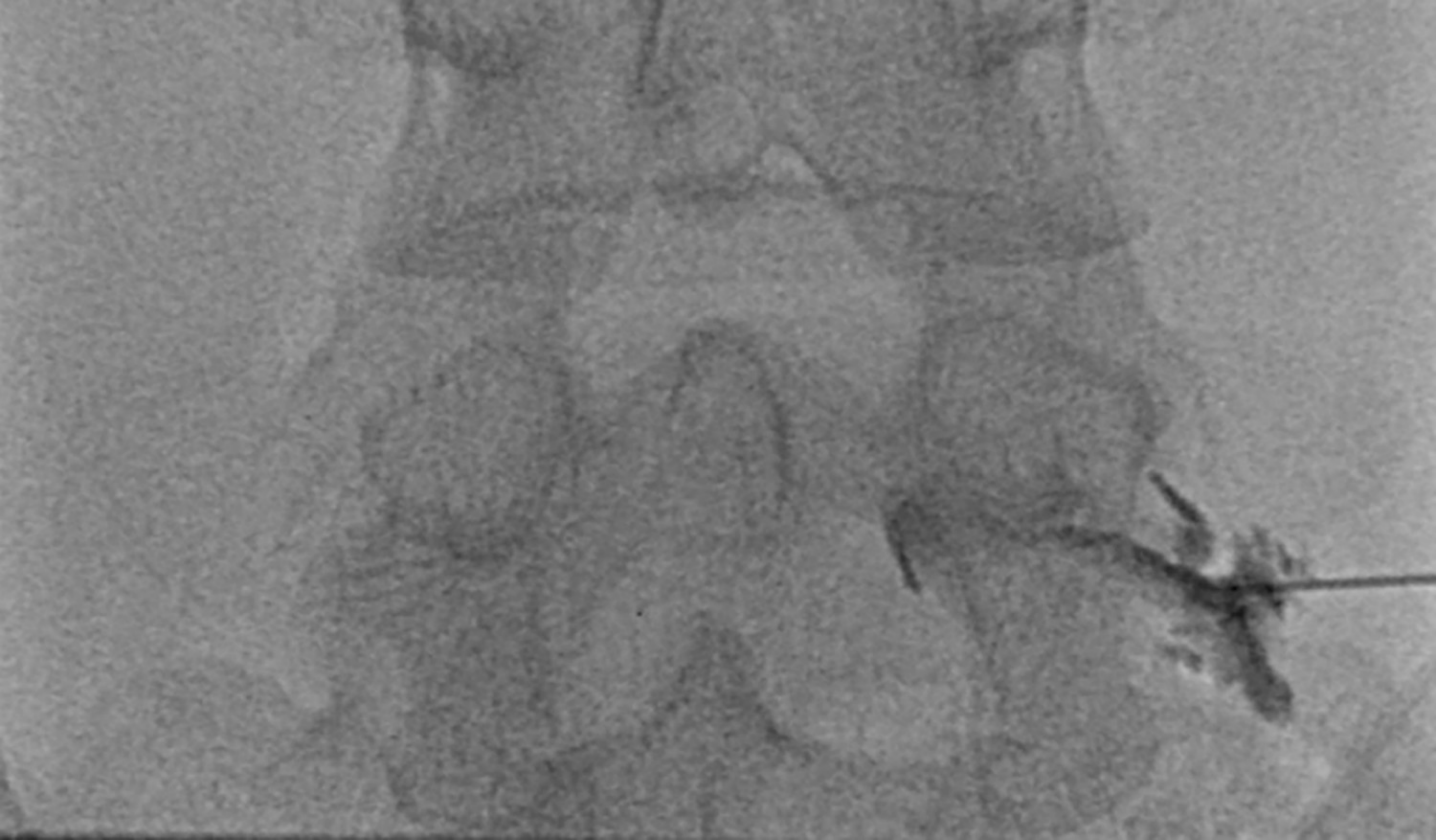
A spot radiograph of a 38-year-old man with radiating pain to the right buttock and lower leg in the L5 dermatome. A magnetic resonance image (not shown) revealed a right central L5-S1 herniated intervertebral disc with compression of the right S1 root. A transforaminal injection was performed at the L5-S1 neural foramen.
